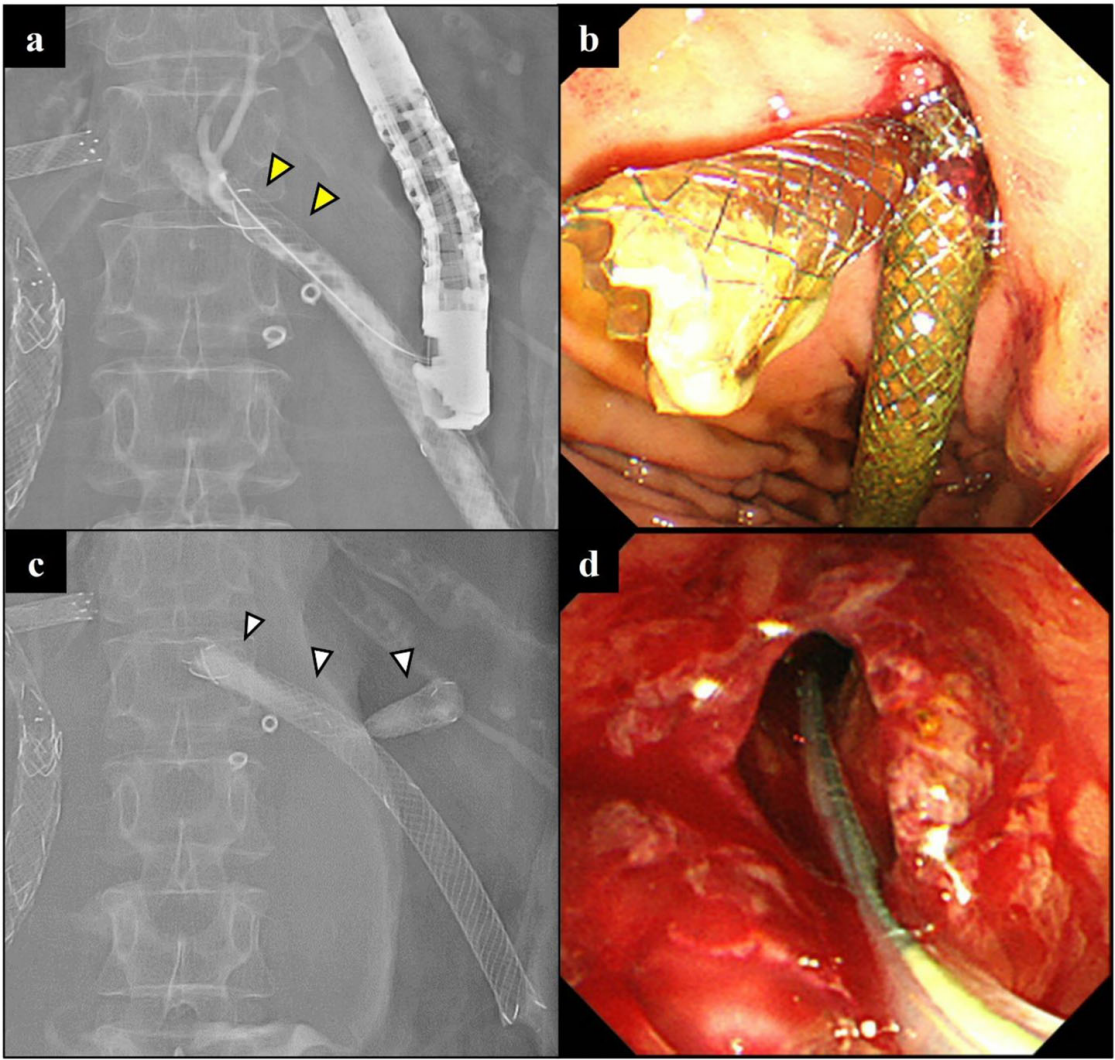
Stent‐in‐stent method via the endoscopic ultrasonography‐guided hepaticogastrostomy route. (a) Six months after the first stent placement, the stent tip has become embedded owing to hyperplasia, making removal impossible (yellow arrowhead). (b) The second stent is placed through the mesh of the first stent. (c) Fluoroscopic image after placing the second stent (white arrowhead). (d) Two months after the second stent placement, both stents are easily removed using grasping forceps.
